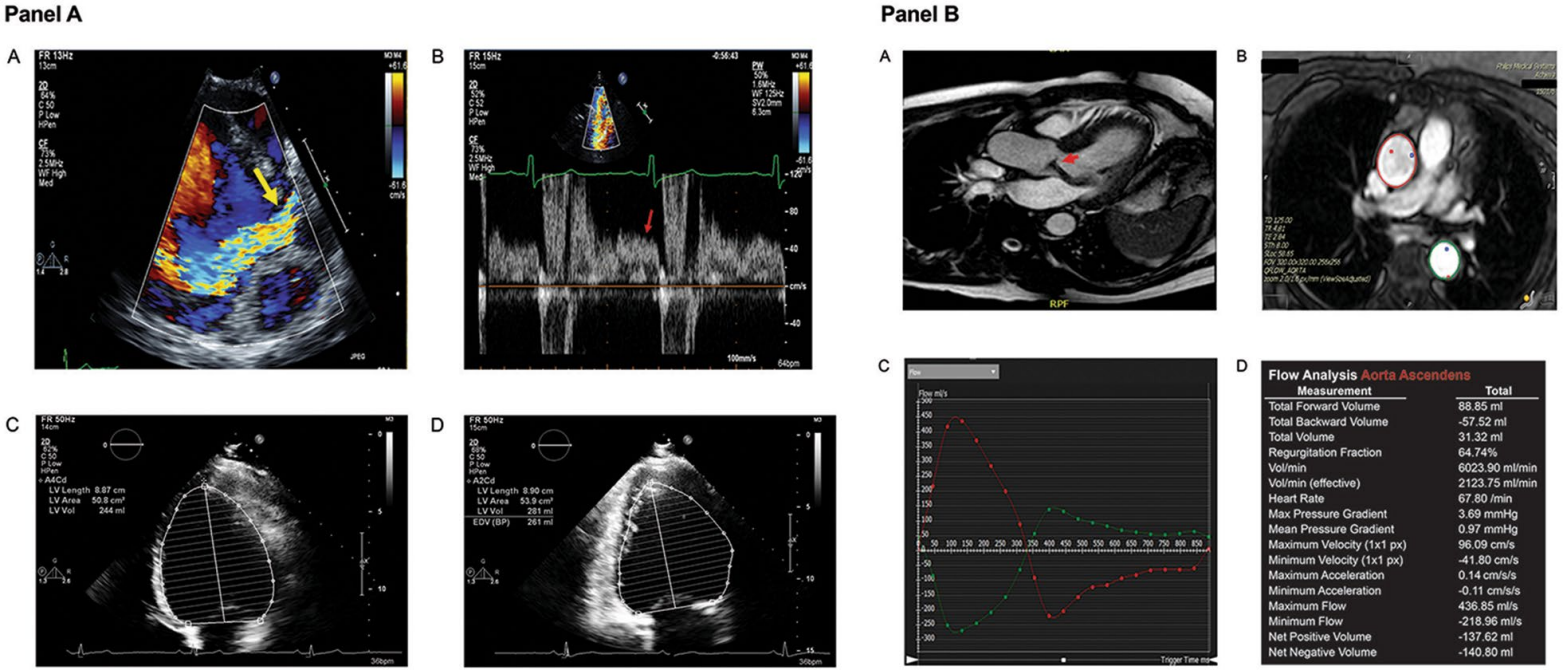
a Assessment of aortic regurgitation (AR) by transthoracic echocardiogram. A Apical 5-chamber view with Color Doppler demonstrating AR (yellow arrow). B Pulse-wave Doppler within the descending aorta with holodiastolic flow reversal (red arrow). C, D Apical 4-chamber and 2-chamber views (respectively) demonstrating traced left ventricular (LV) end-diastolic volume. b Assessment of AR by cardiovascular magnetic resonance (CMR). A Cine balanced steady-state free precession image demonstrating dephasing consistent with AR (red arrow). B Phase-contrast imaging with contours traced for the ascending (red contour) and descending (green contour) aorta. C Measured anterograde and retrograde flow of the ascending (red line) and descending (green line) aorta with holodiastolic flow reversal in the descending aorta (green arrows). D Measurements obtained from flow analysis
